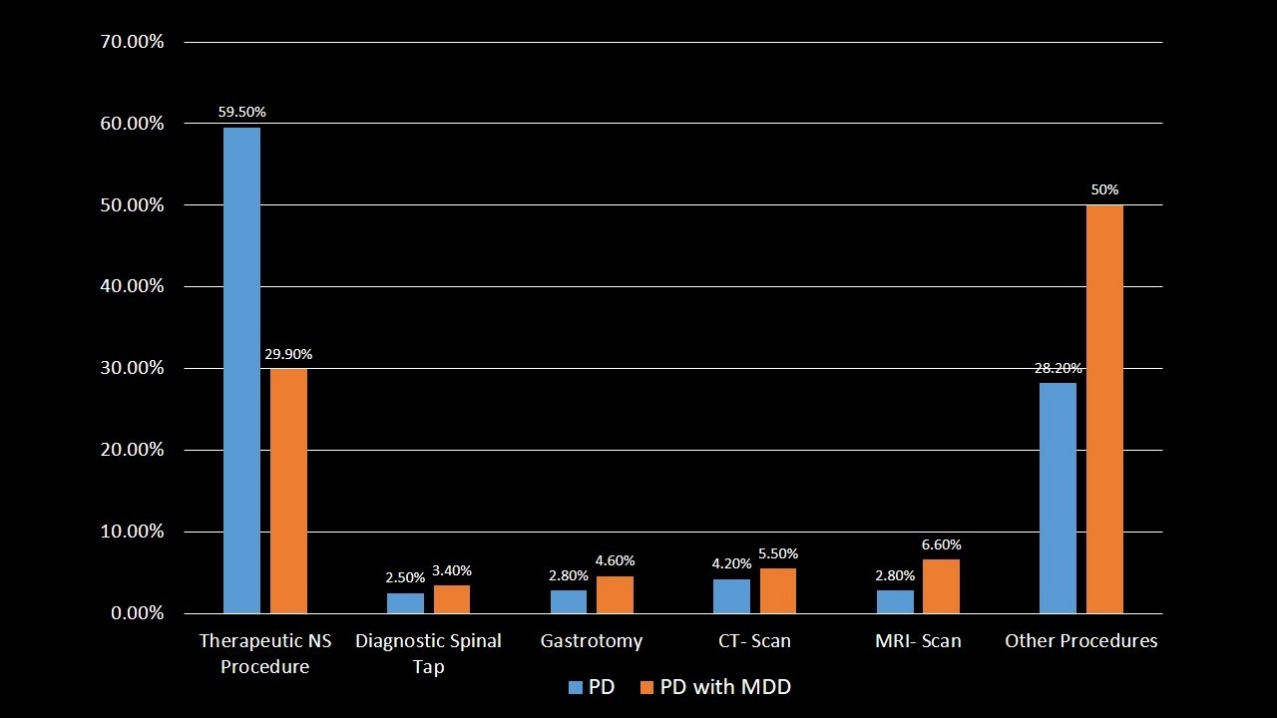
Procedures in Parkinson's disease and Parkinson's disease with major depressive disorder patients. PD: Parkinson's disease; MDD: Major depressive disorder; NS: Nervous system; CT: Computed tomography; MRI: Magnetic resonance imaging.
